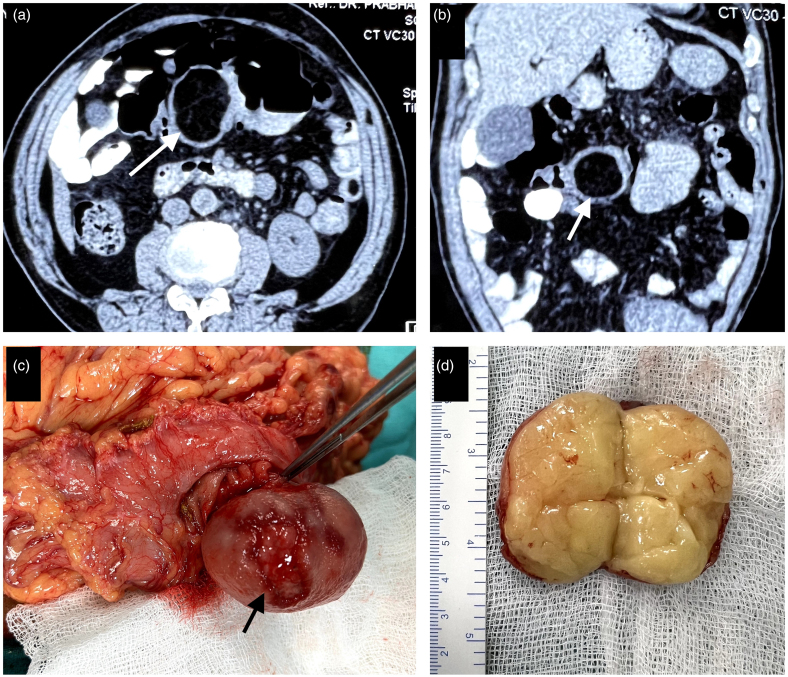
(a and b) Abdominal CT scan images showing lipoma in transverse colon with characteristic fat equivalent lesion density (white arrow). (c) Intraoperative image showing a submucosal lesion with ulcerated mucosa (black arrow). (d) Cut-section examination showing a fat-containing capsulated tumour.
